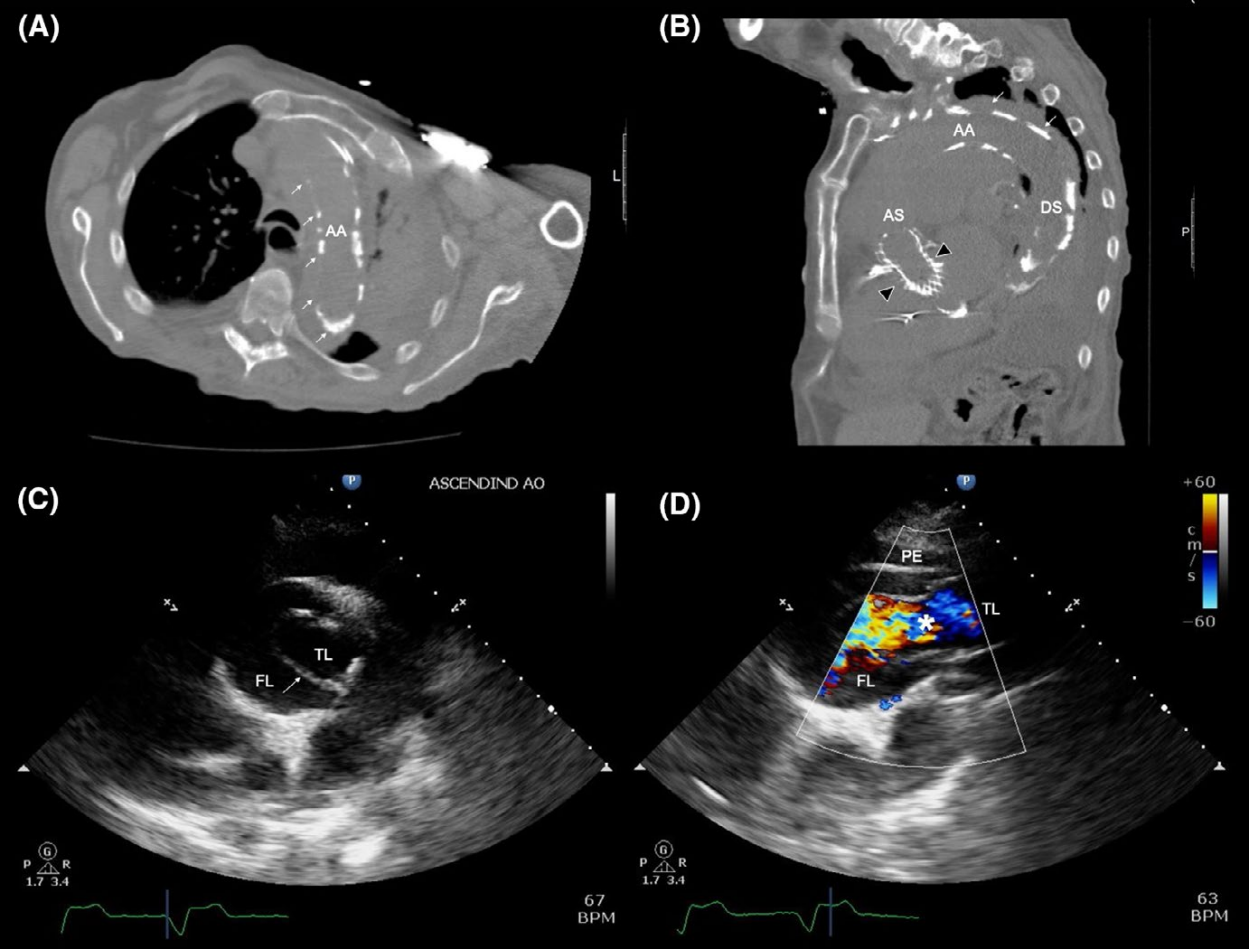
(A) Axial and, (B) Sagittal view of an unenhanced computed tomography (CT) of the chest showing a prominent aneurysmal dilation of the thoracic aorta with severe atherosclerotic vascular calcifications and internal displacement of mural calcifications toward the aortic lumen (arrows), suggestive of a Stanford type A aortic dissection; and demonstration of a prosthetic aortic valve in appropriate location (black arrowheads). AA, aortic arch; AS, ascending aorta; DS, descending aorta. Transthoracic echocardiogram, (C) parasternal short‐ axis view, and (D) parasternal long‐ axis view showing a severely dilated ascending aorta with a complex intimal flap (arrow) that divides the aorta into two, true and false, lumen; and (D) demonstrates the true lumen by color Doppler (asterisks) and a pericardial effusion. FL, false lumen; PE, pericardial effusion; TL, true lumen
